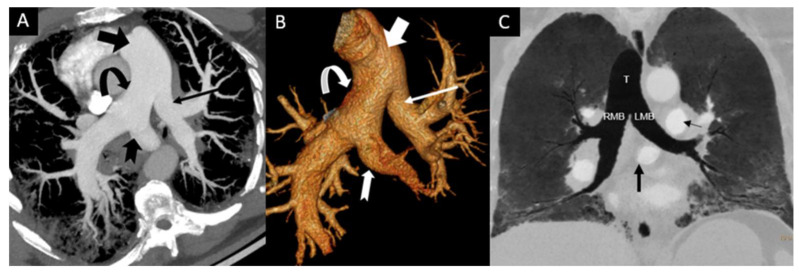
Incidental finding of PALPA in a 51-year-old male with acute shortness of breath. Contrast enhanced chest CT obtained to rule out pulmonary embolism demonstrates that a duplicated left pulmonary artery with a small left pulmonary artery (thin arrow) to left upper lobe was arising from the main pulmonary artery (bold arrow) and an additional anomalous left pulmonary artery to the left lower lobe (notched arrow) was arising from the proximal posterior part of the right pulmonary artery (curved arrow) on axial oblique reconstructed Maximum Intensity Projection (MIP) image (A) and Volume Rendered reconstructed image (B). There was no associated congenital heart disease or tracheobronchial stenosis. Coronal Minimum intensity projection (MinIP) (C) reconstructed image from same patient shows that the course of anomalous LPA branch (PALPA, bold black arrow) is caudal to the carina and is inferior anterior and parallel to the left main bronchus (LMB). There was no tracheal (T), right main (RMB) or left main bronchial (LMB), or esophageal compression. Note that left upper lobe and the lingula were supplied by the proximal left pulmonary artery (thin black arrow) which arises in the usual position from the main pulmonary artery (MPA). Note peripheral reticular opacities and ground glass opacities in both lungs related to known non-specific interstitial pneumonia (NSIP).
